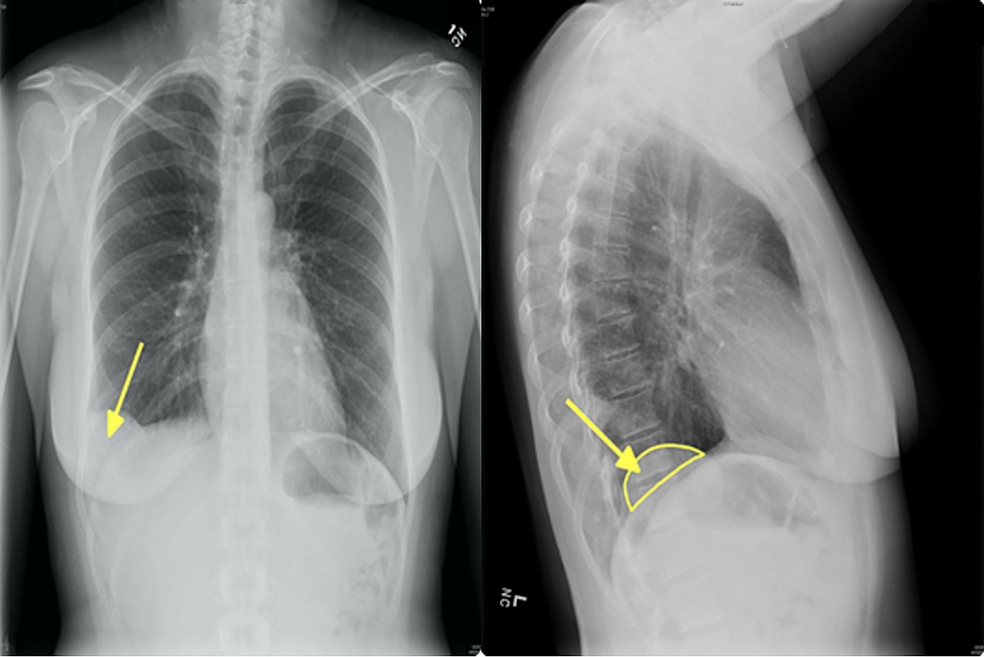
Chest radiograph anteroposterior and lateral views demonstrating a mass in the right costophrenic angle (arrow) and diaphragmatic eventration on the left (semicircle).
